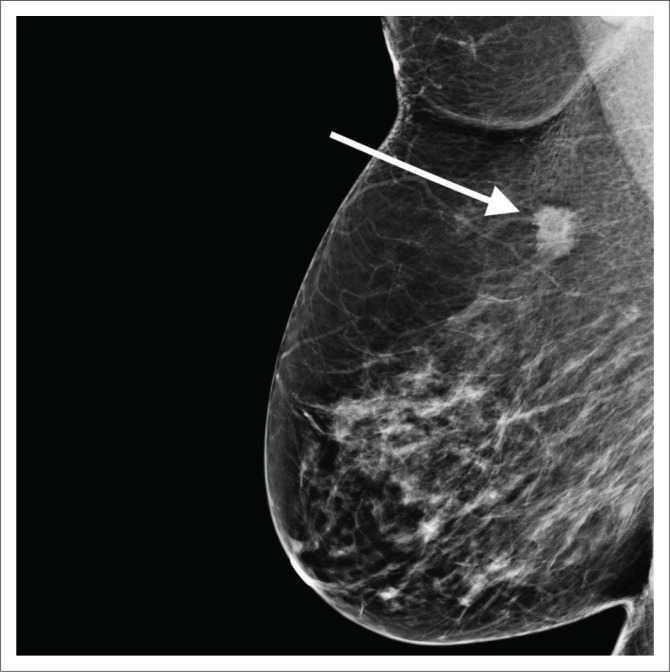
Mammogram (RMLO) in a 70-year-old patient shows a solitary spiculated mass in the upper breast (white arrow). There is clearly no invasion of surrounding tissue and absence of dense fibroglandular tissue that may be obscuring multifocal or multicentric disease. Biopsy revealed a low-grade luminal A cancer. This patient was not a candidate for pre-operative breast magnetic resonance imaging. RMLO, Right medio-lateral Oblique view.
